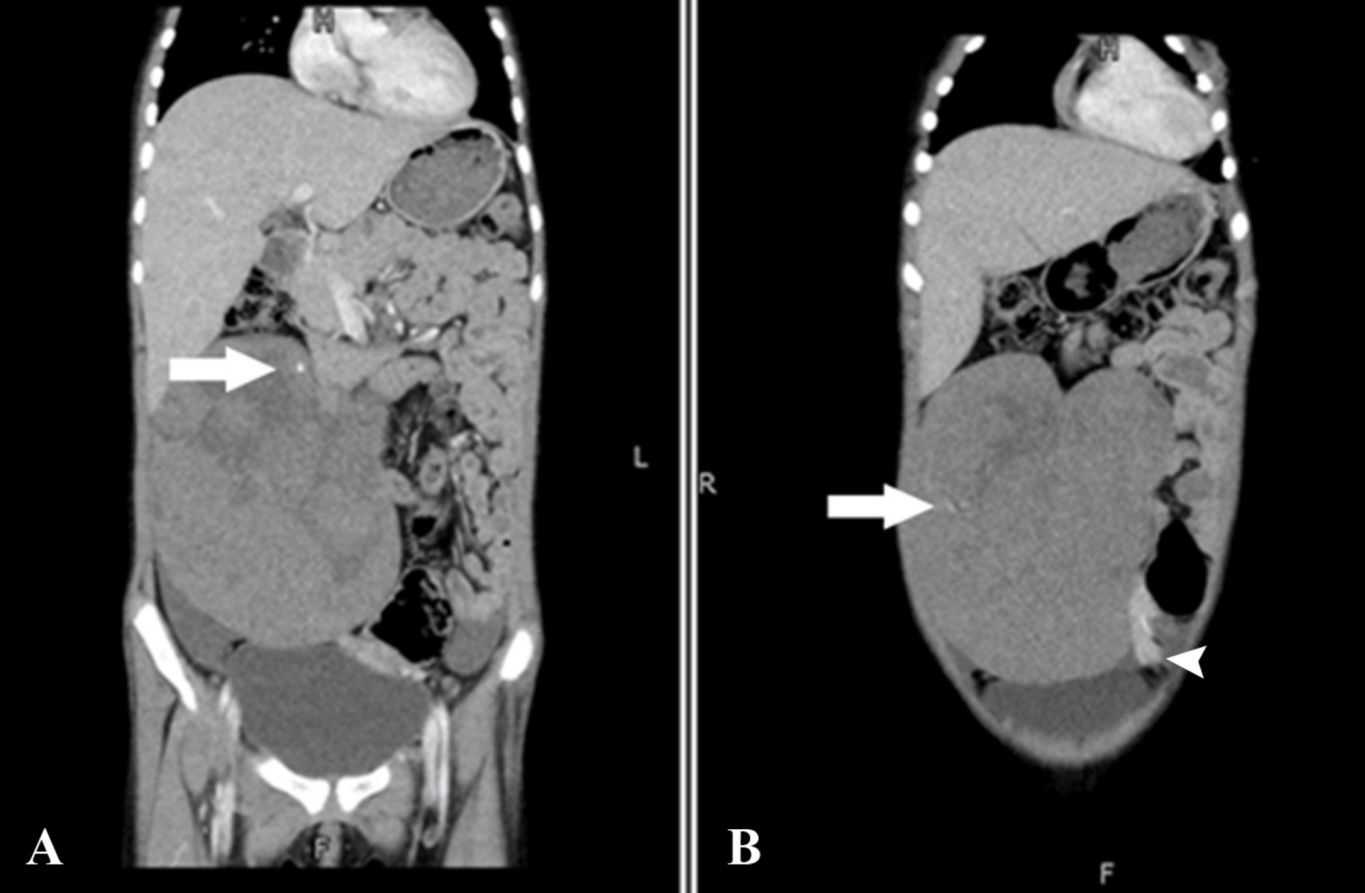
Computed tomography of the abdomen and pelvis coronal sections Speckled calcifications were noted in the mass (arrows). Partially visualised twisted vascular pedicle also seen (arrowhead).
